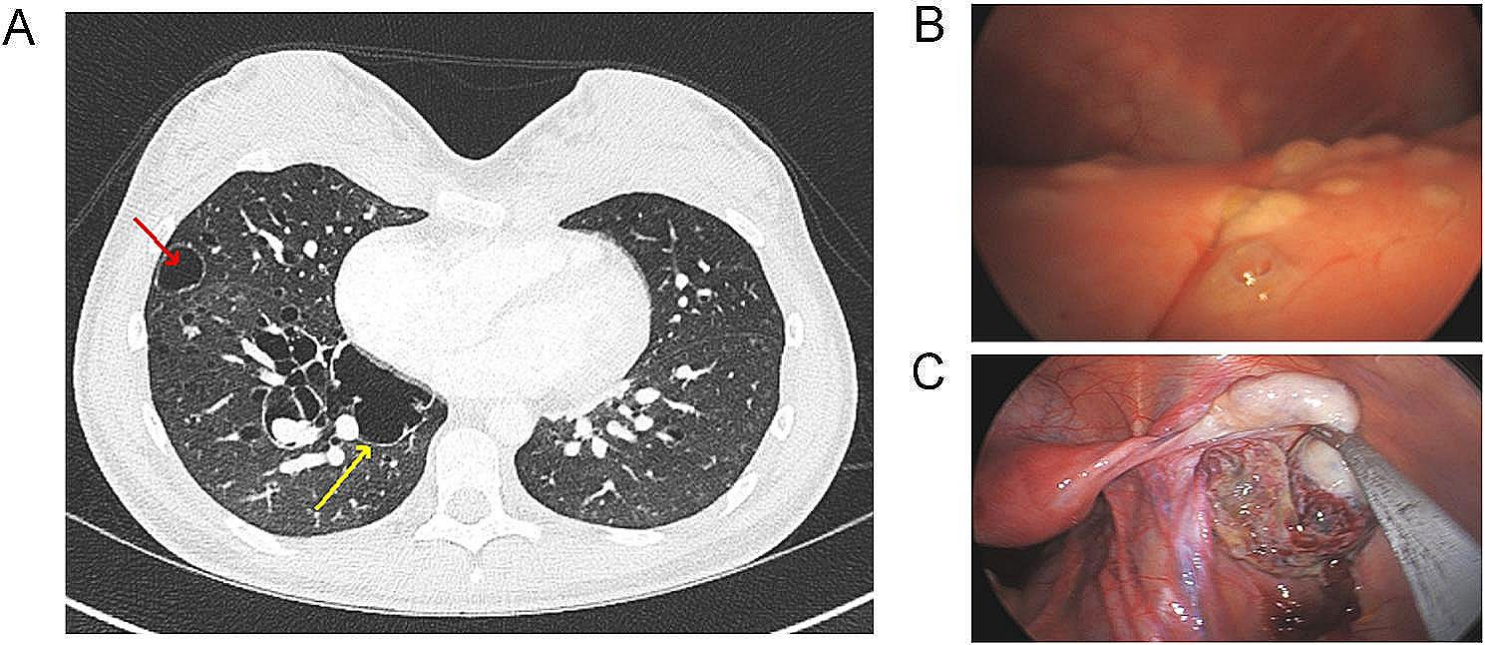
Computerized tomography (CT) scan identified multiple thin-walled cysts throughout both lungs (A, arrows indicate cysts). Thoracoscopic examination showed that the lungs surface was notable for multiple cystic lesions and nodules (B). Laparoscopy examination demonstrated that the right ovary and tube appeared inflamed with attached partially necrotic hemorrhagic cyst (C)
